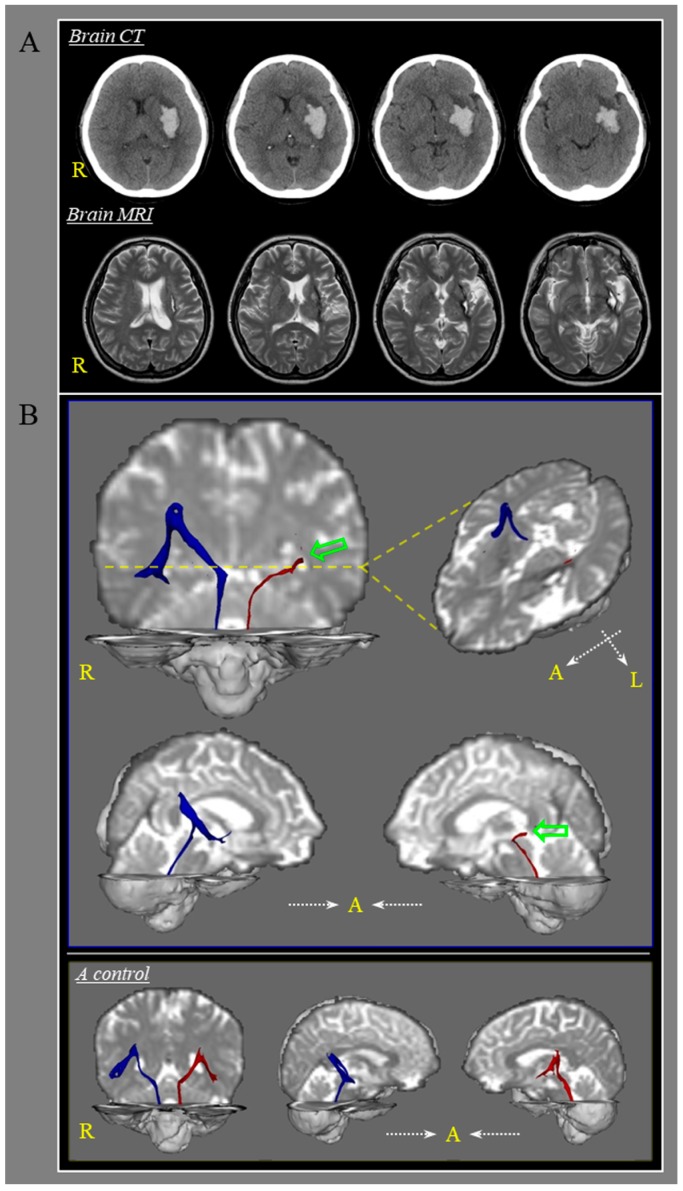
A 51-year-old female patient underwent conservative management for a spontaneous intracerebral hemorrhage (ICH) in the left basal ganglia (mainly affecting the internal capsule and insular cortex) (A). When she visited the rehabilitation department of the university hospital at two years after the ICH onset, she reported that moderate dizziness (mainly, light-headedness) had started after the ICH onset. She mentioned that her dizziness had decreased slightly over time. Physical examination by an otorhinolaryngologist revealed no abnormalities in the vestibular system of either ear. She also exhibited mild right hemiparesis; however, her cognitive function was within the normal range (Mini-Mental State Examination score, 28, cutoff value: <25). Brain magnetic resonance images were obtained two years after onset and revealed leukomalactic lesions in the left basal ganglia and the subcortical white matter of the insular cortex (A). On diffusion tensor tractography, the patient’s core vestibular projection in the left hemisphere (red) showed discontinuation (green arrows) at the basal ganglia level compared with that of the patient’s right core vestibular projection (blue) and those of a normal subject (B). The patient and a normal subject (50-year-old female) provided written informed consent for participation in this study, and the study protocol was approved by the Yeungnam university hospital institutional review board (YUMC-2019-06-032, 28 June 2019). A: anterior, R: right, L: left, Yellow dotted line: level of lesion.
